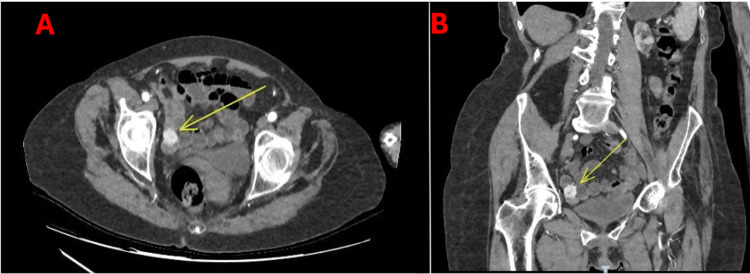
Contrast-enhanced (arterial phase) computed tomography (CT) scan before perforation A: Axial view; B: Coronal view. The yellow arrow indicates a 2 cm avidly enhancing small bowel mass initially suspected to be a GIST. GIST: Gastrointestinal stromal tumor
